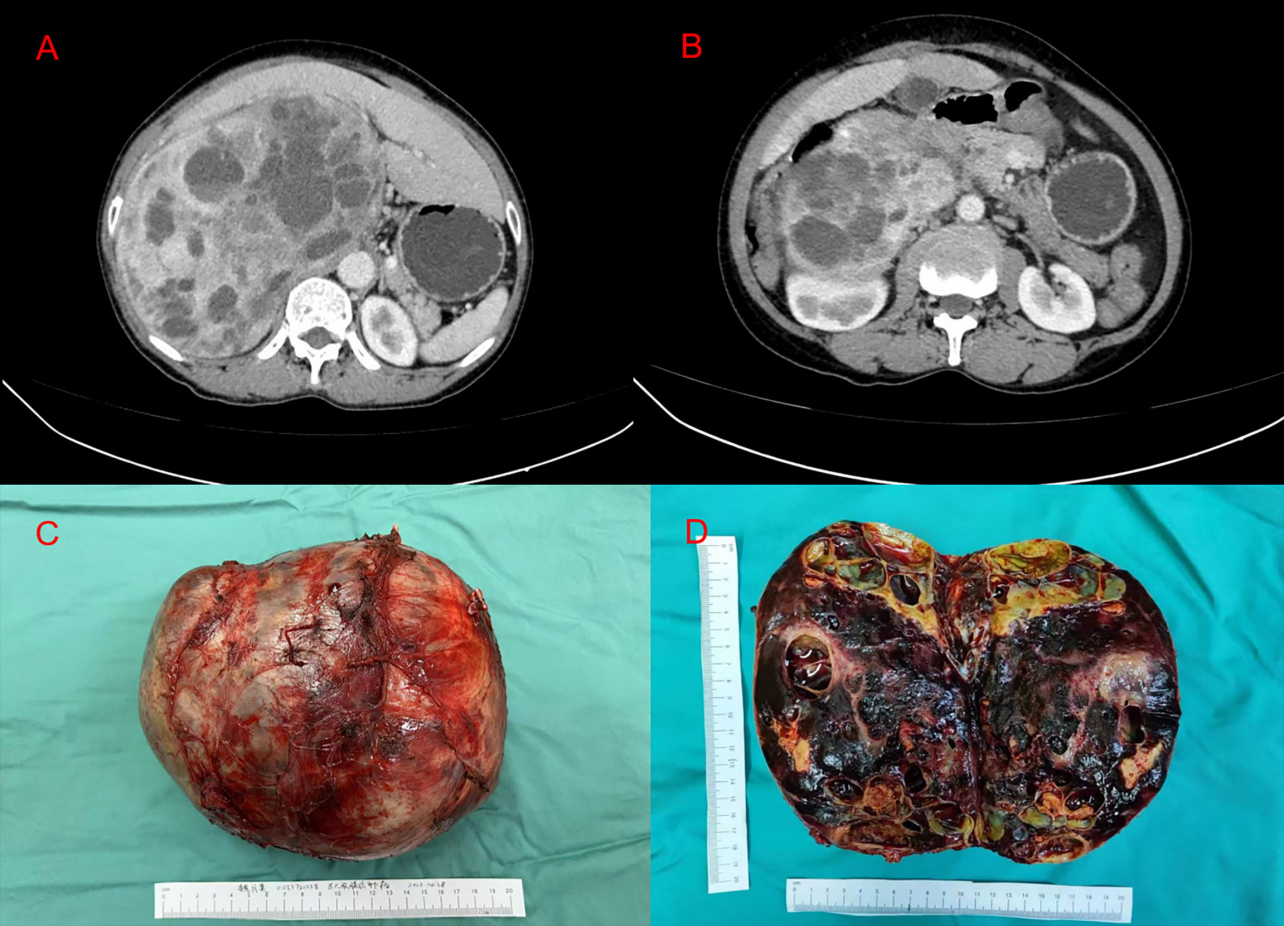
(A, B) Abdominal enhanced computed tomography imaging representing the huge tumor between the right lobe of the retroperitoneal liver and the right kidney. (C) A macroscopic image of the post-section cystic pheochromocytoma. (D) Excised tumor, measuring 20 × 15 × 10 cm.
